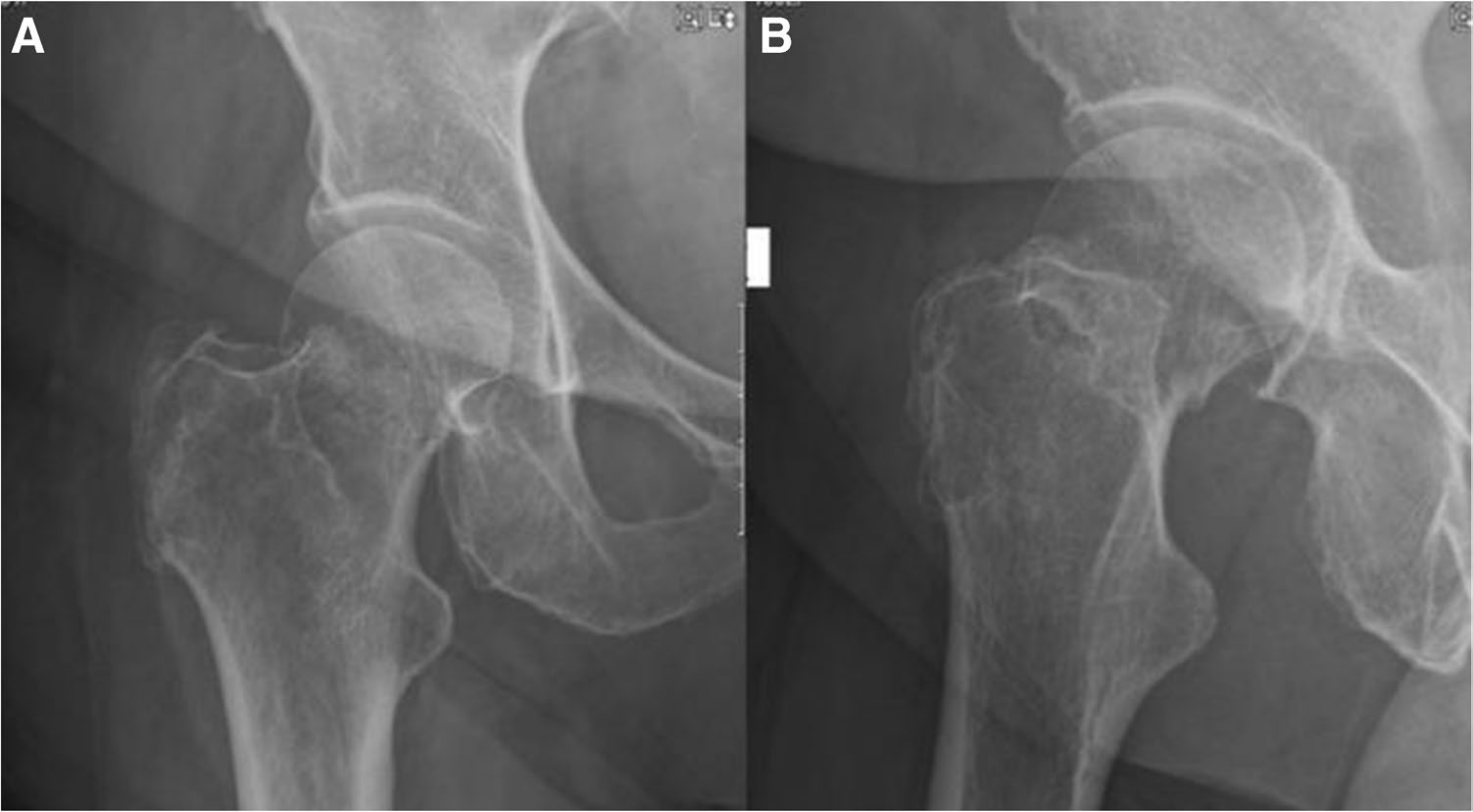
A, B AP and lateral radiographs showing intracapsular femur fracture of a 77-year-old female, which was admitted after a fall from standing height. Patient walks with no aids before the injury; her medical history consist of hypertension and dyslipidaemia
